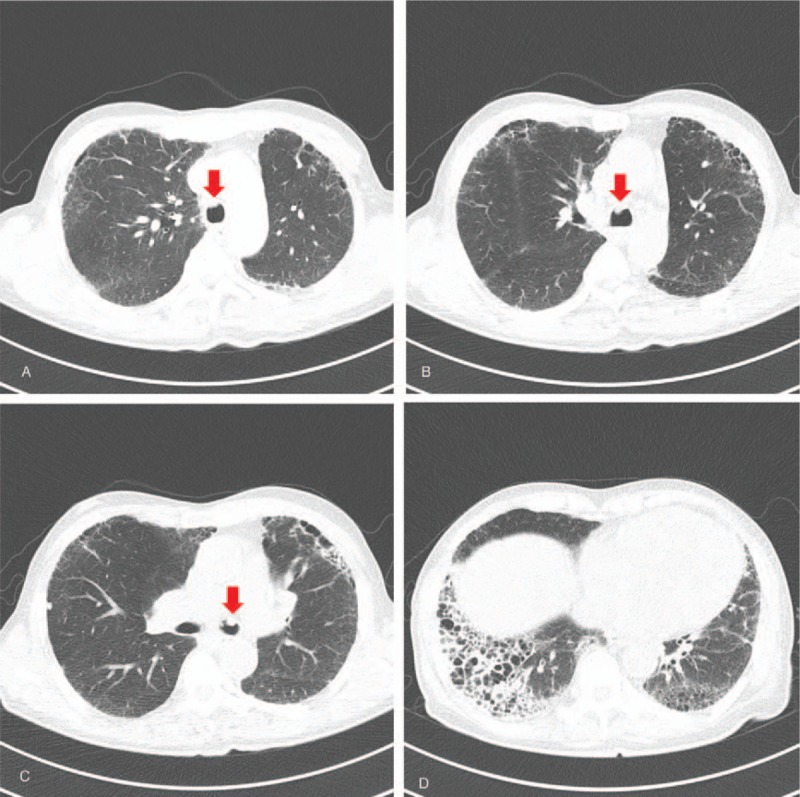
(A–C) Chest CT showed a mass at the distal trachea extending to carina and left main bronchus. (D) Chest CT showed honeycomb change and traction bronchiectasis at peripheral portion of both lower lung fields. CT = computed tomography.
